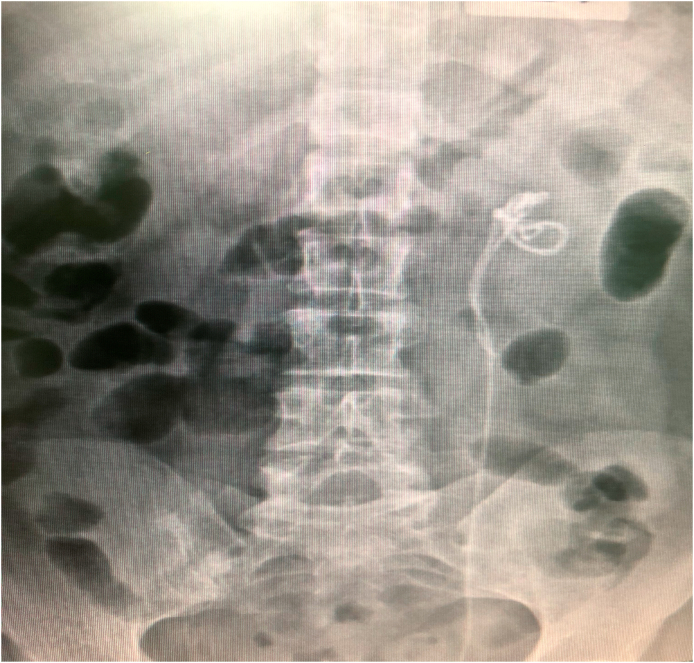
X-ray of the left kidney with two double J stents placed in it.
